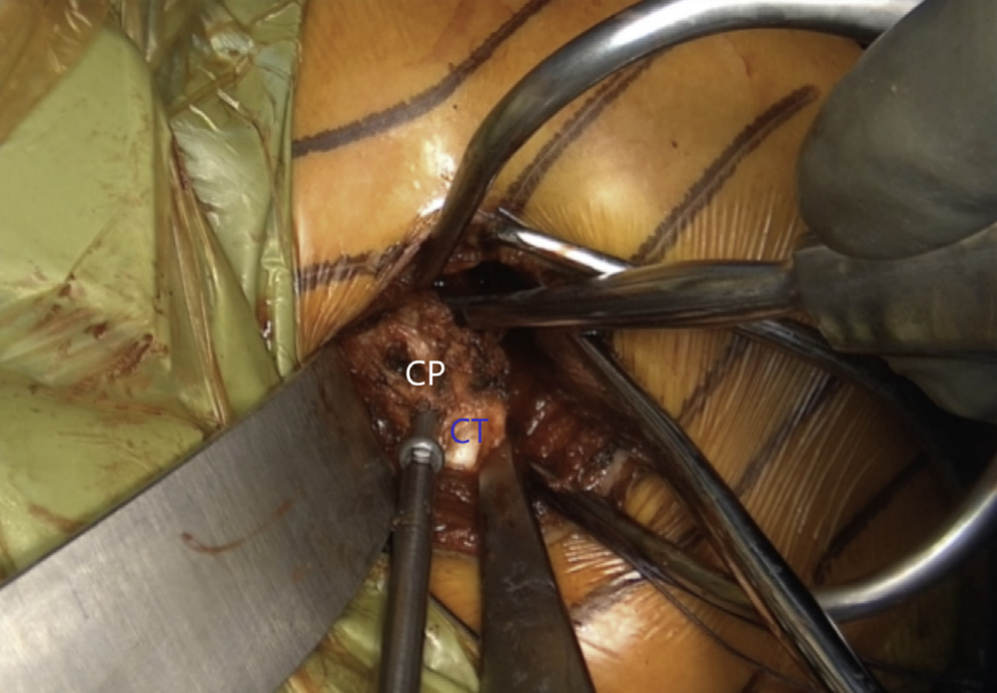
Left shoulder of a patient placed in a semi beach-chair position. The coracoid process (CP) is placed at the prepared AGN surface. First, the inferior screw is introduced for preliminary fixation of the coracoid process so that the graft can still slightly rotate around the screw. (CT, conjoined tendon.)
